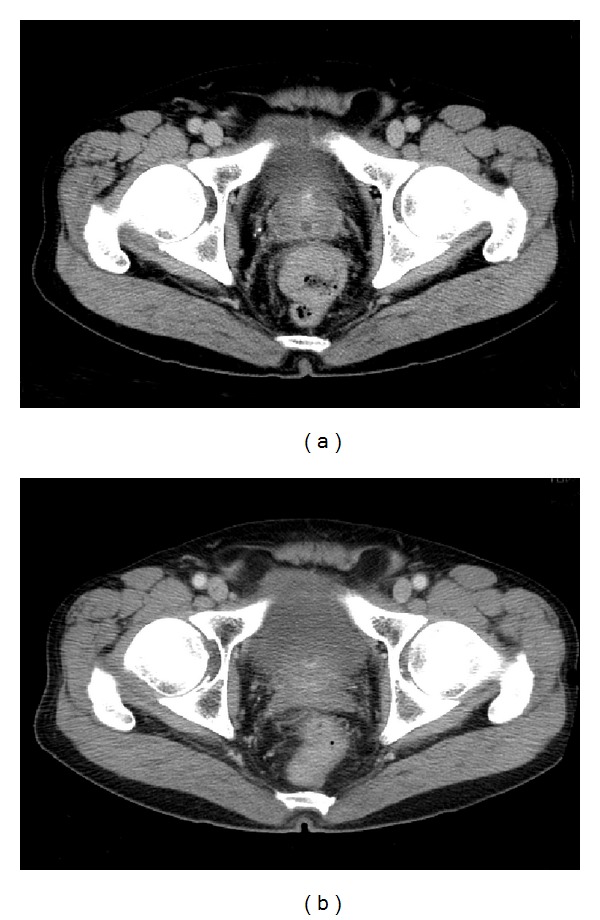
Abdominal computed tomography (CT) findings both (a) before and (b) after CRT.
